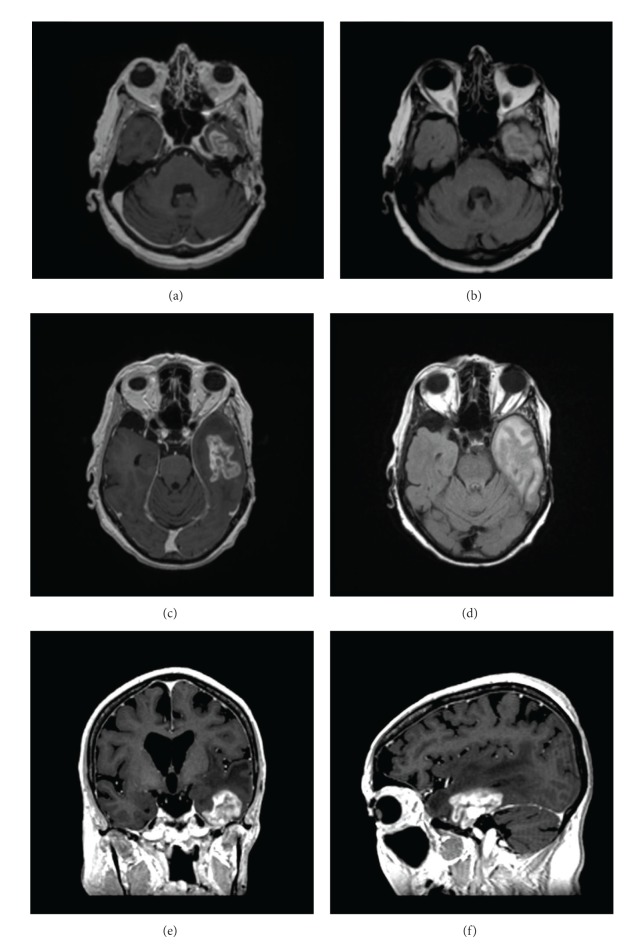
Axial slices of T1 postcontrast (a) and T2 FLAIR (b) MRIs are shown, corresponding to the radiation plan seen in Figure 1. Additional axial T1 postcontrast (c), axial T2 FLAIR (d), coronal T1 postcontrast (e), and sagittal T1 postcontrast (f) slices depict an irregularly enhancing mass lesion in its greatest dimensions, involving the left temporal lobe tip. The lesion measured 4.6 cm in the greatest dimension with significant surrounding edema. There was evidence of temporal bone resection, consistent with prior resection of the left parotid gland.
